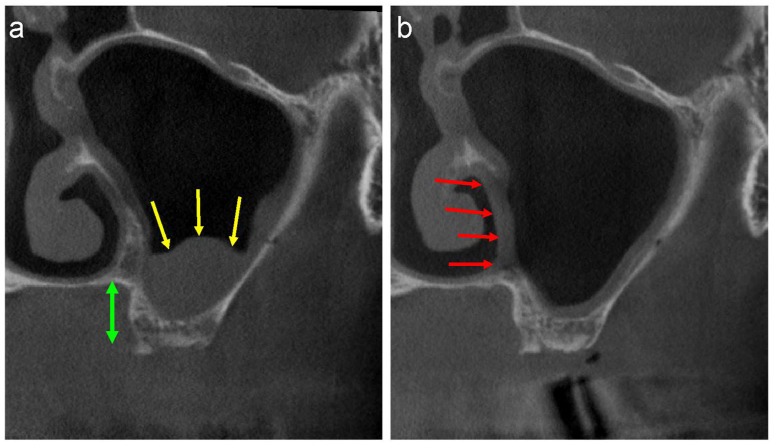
(a) The buccolingual section of the CT image shows well-defined, faintly radiopaque antral lesions (yellow arrows), and the distance between the alveolar crest and bottom of the nasal cavity is short (green up-down arrow). (b) A bone defect in the medial wall is observed following the removal of the lesion by the ENT surgeon (red arrows).
